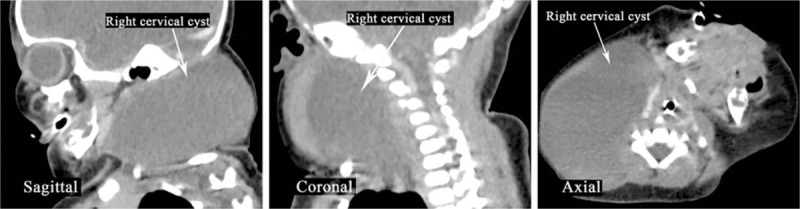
Fetal Computed tomography (CT) images after Birth. CT revealed a 4.0- × 6.1- × 4.8-cm cystic lesion without obvious strengthening in right neck; the upper part of the lesion was adjacent to the auricle and the lower part reached the right supraclavicular fossa.
